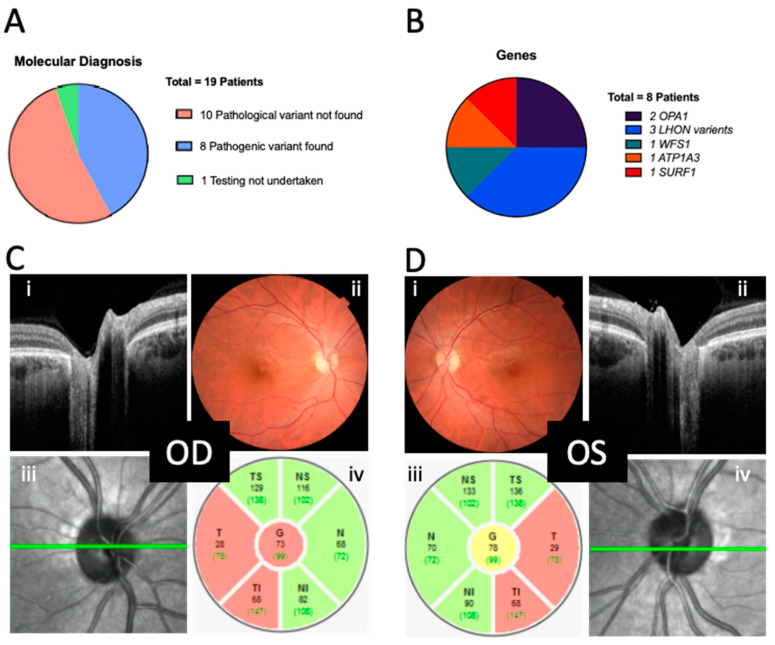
Molecular diagnoses. (A) Proportion of patients who underwent genetic testing and received a molecular diagnosis. (B) Identity of gene where pathological variant was found in those receiving a diagnosis. (C,D) Montage of images from patient B (OPA1c.1608+1G>A, Table 1) who presented with gradually progressive optic neuropathy from 14 years of age. Visual acuity at this time was R0.60/L0.70LogMAR with temporal disc pallor noted clinically, with ganglion cell dysfunction noted on electrophysiological investigation. MRI and other investigations for secondary causes were non-contributory and so genetic testing proceeded (Supplementary Table S1). (Ci,Dii) Optical coherence topography views of the disc in cross section and (Ciii,Div) en face. (Cii,Di) Colour fundus photos. (Civ,Diii) Peripapillary retina nerve fibre layer thicknesses; colour coding indicates comparison to normative database (Heidelberg, European)—green = within normal limits, red = below normal limits. Note temporal thinning in both eyes: N—nasal, I—inferior, T—temporal, S—superior. OD—Oculus dexter, right eye; OS—Oculus sinister, left eye.
